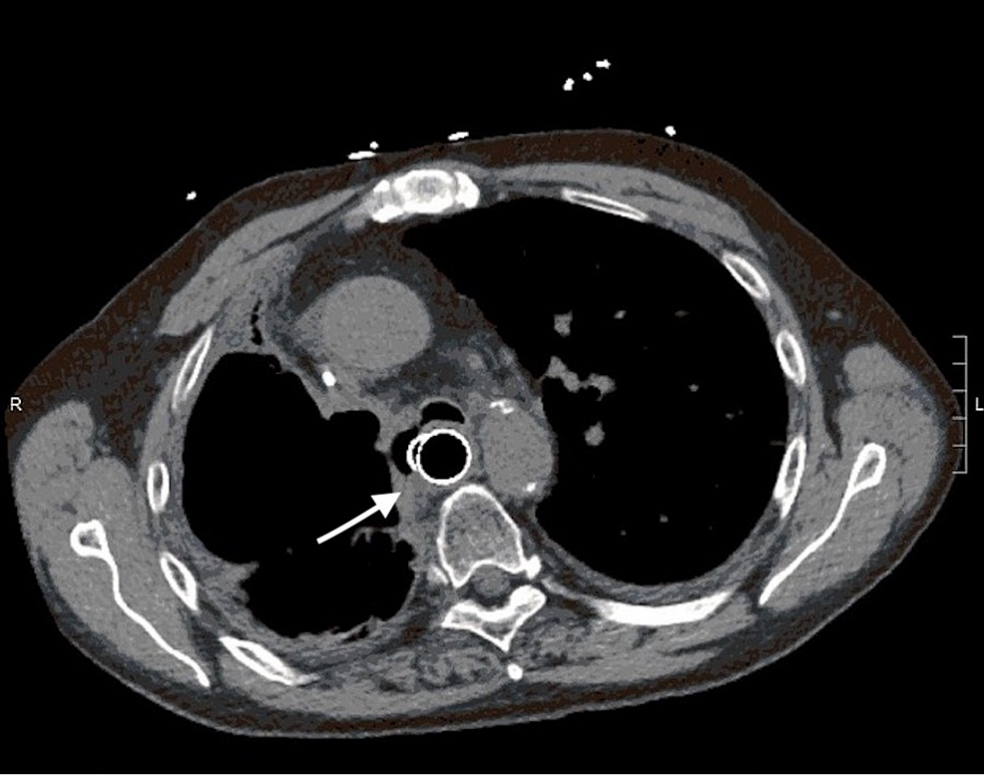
Axial computed tomography showing the parenchymal-esophageal fistula after closure (arrow)
